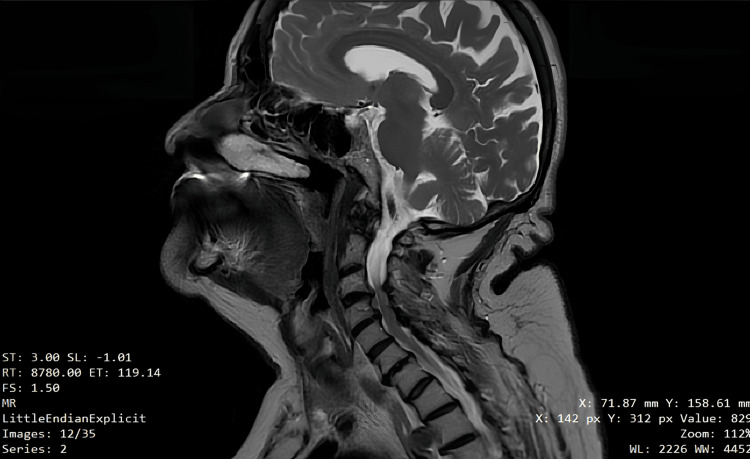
Five-month magnetic resonance imaging of the neck.
